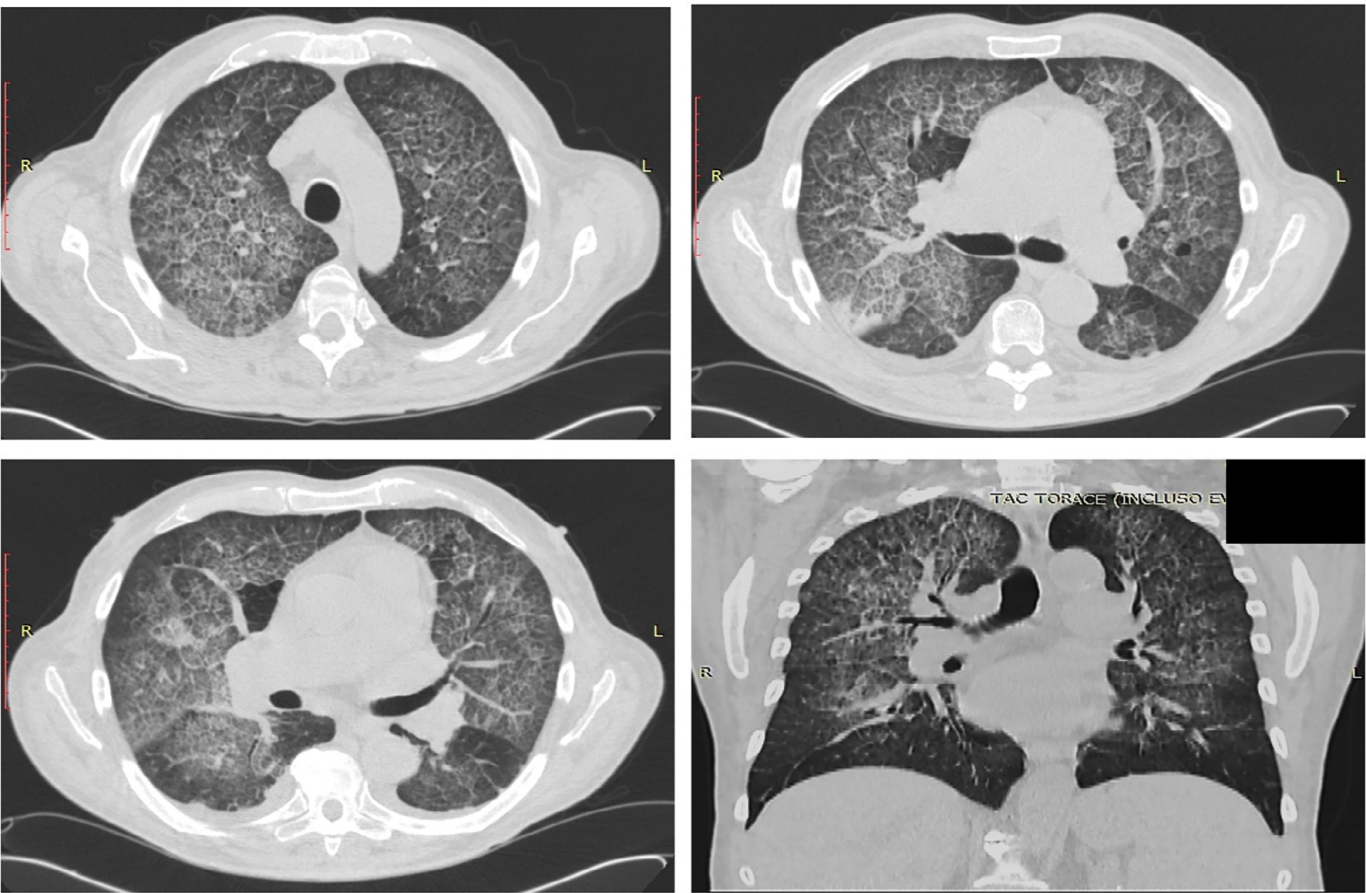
Chest computed tomography scan at admission to hospital.
